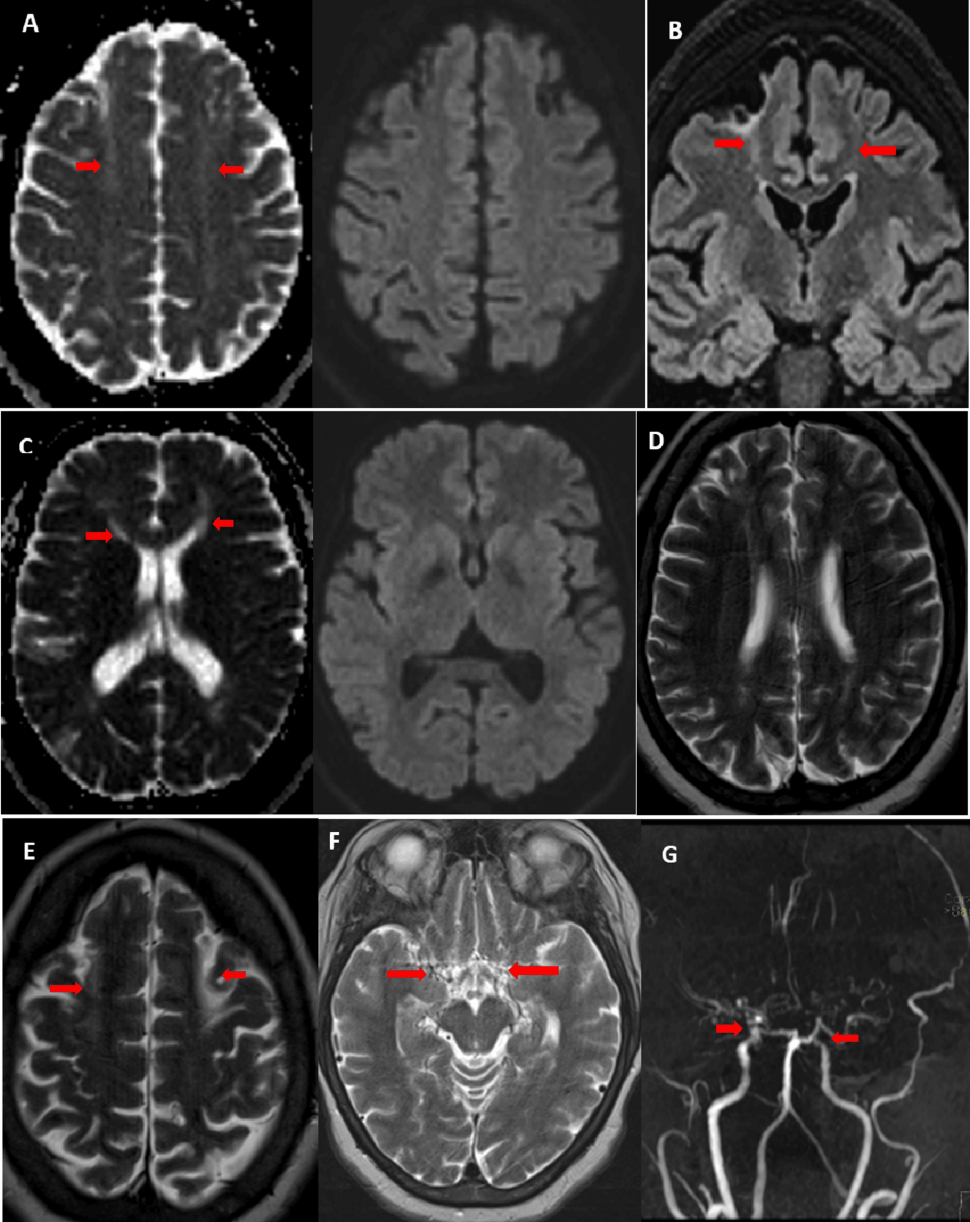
Cerebral MRI showing bilateral frontal cortico-subcortical hyperintensities on apparent diffusion coefficient (ADC) with normal diffusion (A, C), fluid-attenuated inversion recovery (FLAIR) (B), and T2 (D, E) sequences. Magnetic resonance angiography (MRA) of the circle of Willis (G) demonstrates internal carotid artery stenosis bilaterally, with absence of visualization of the middle cerebral arteries (MCA) and anterior cerebral arteries (ACA), replaced by a moyamoya collateral network visible on the T2 sequence (F).
